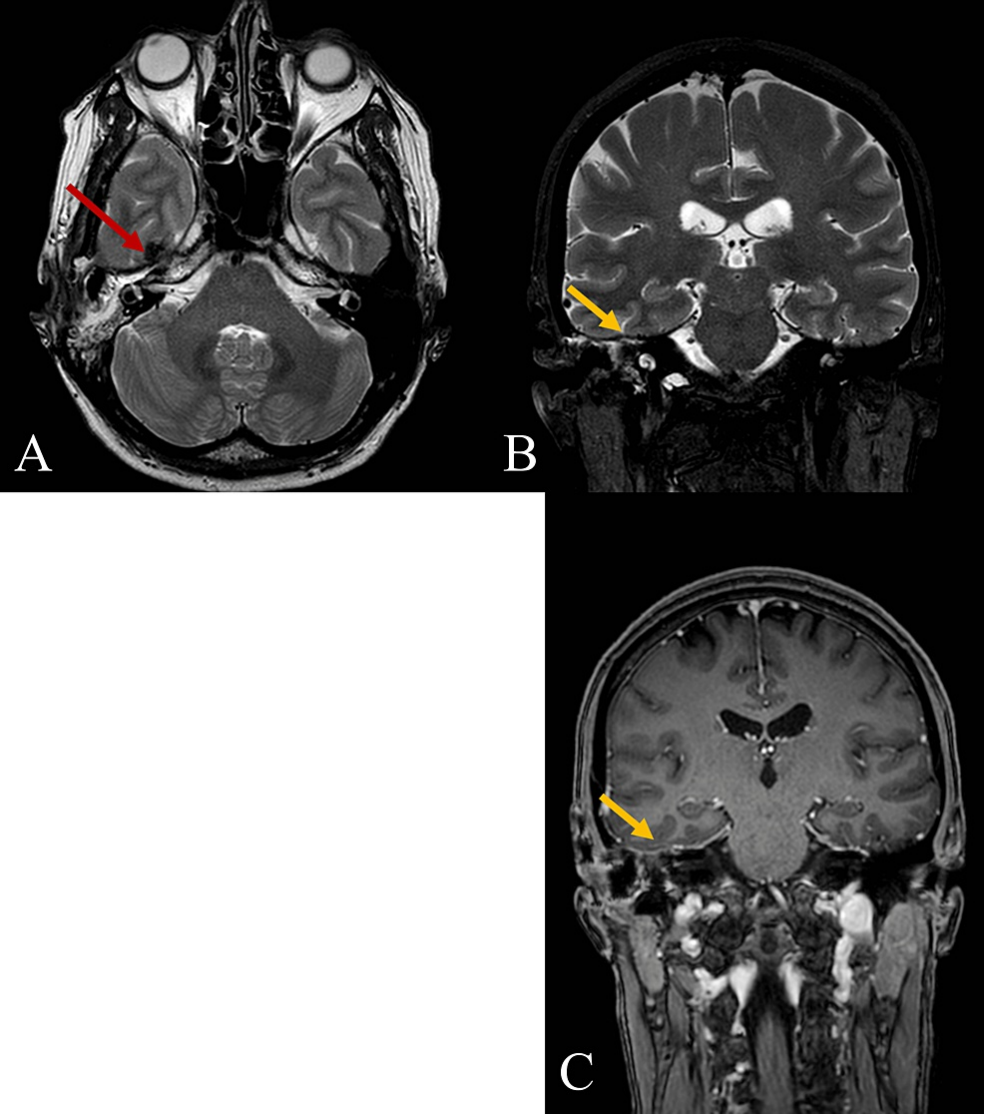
Postoperative MRI in T2-weighted axial (A), coronal (B) and T1-weighted coronal (C) views, the latest follow-up in 2023 Postoperative changes (red arrow) after the surgical tumor removal and duroplasty is seen. No residual tumor in the previously affected location (yellow arrows) is visible.
